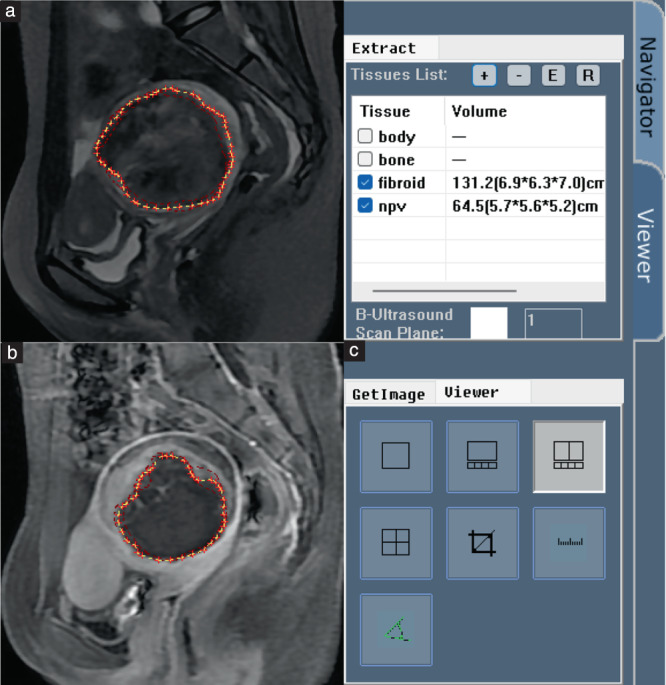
MicroSea 3D Image Processing Software was used to determine myoma volume and volume of non‐perfused areas using a layer‐by‐layer process. (a) Myomas were traced manually on T2‐weighted magnetic resonance (MR) images. (b) Non‐perfused areas were traced on contrast‐enhanced MR images. (c) Software calculated the three‐dimensional volume.
